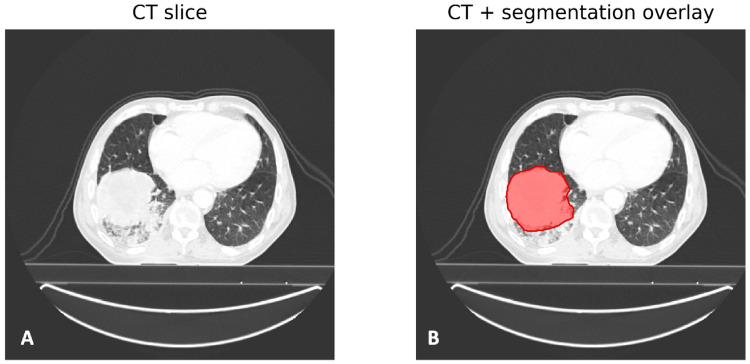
CT slice (A) with corresponding tumor segmentation overlay (B).
